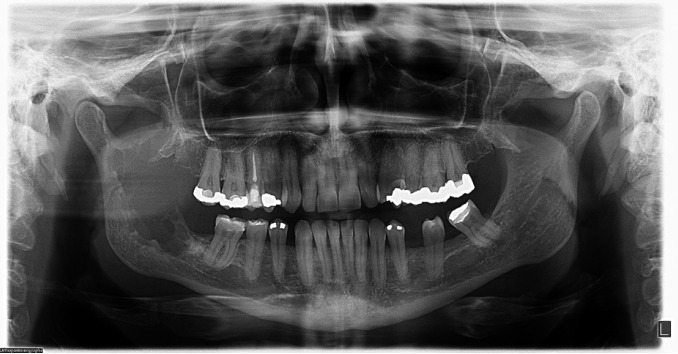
Panoramic radiograph revealing a multilocular radiolucent destructive lesion in the right mandibular ramus.
